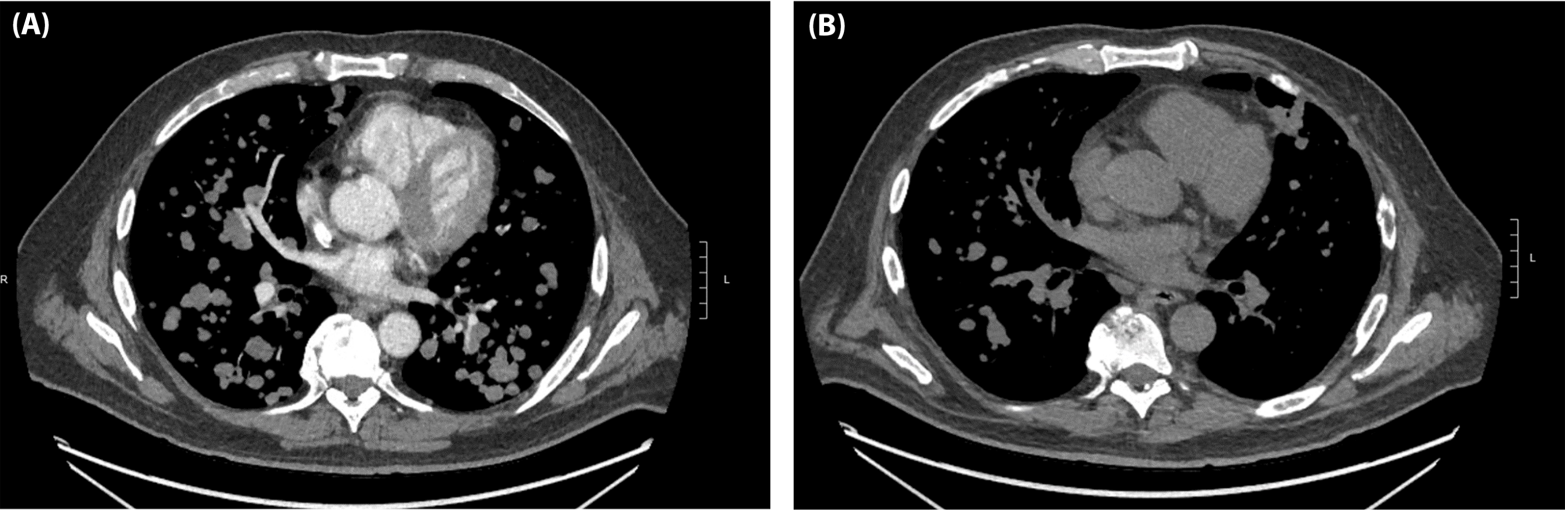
A 52-year-old man with BRAF-wildtype, metastatic melanoma with lung, liver, spleen, and brain involvement who received dose 1 of ipilimumab/nivolumab on 11/15/19 and dose 2 on 12/03/19. (A) Baseline CT chest on 11/06/19 and (B) CT chest on 12/17/19.
